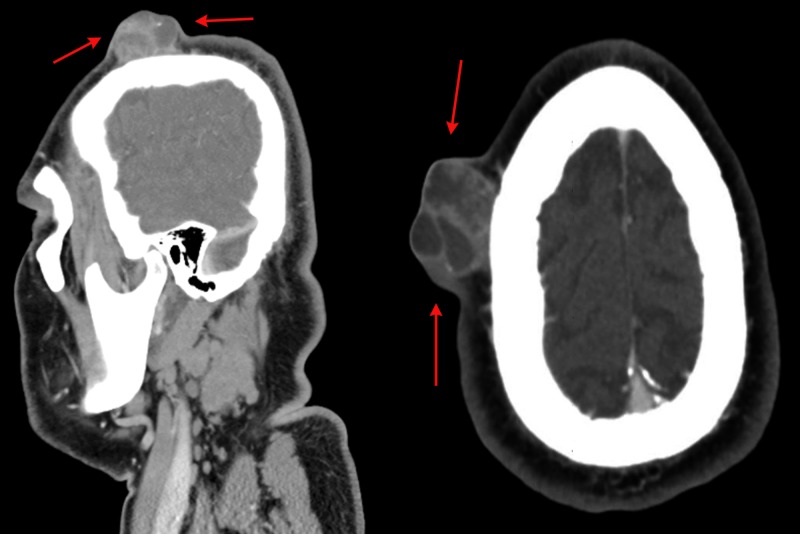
Computed tomography scan showing a lobulated mass lesion on the scalp (red arrows)
